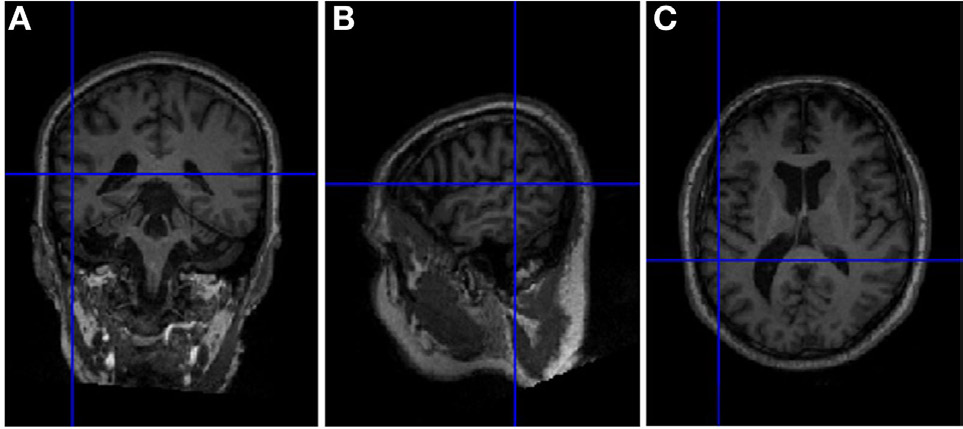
Localization on our patient’s T1 magnetic resonance imaging (MRI) sequence of the left temporoparietal junction region targeted in the treatment of auditory hallucinations in schizophrenia patients as the one we report. This region corresponds to the MNI coordinates *x* = −55, *y* = −41, *z* = 11. As it can be observed in this figure, it is located at the posteriori end of the sylvian fissure, ventral to the supramarginal gyrus. See coronal (A), sagittal (B), and axial (C) MRI views of the location for the transcranial magnetic stimulation target.
